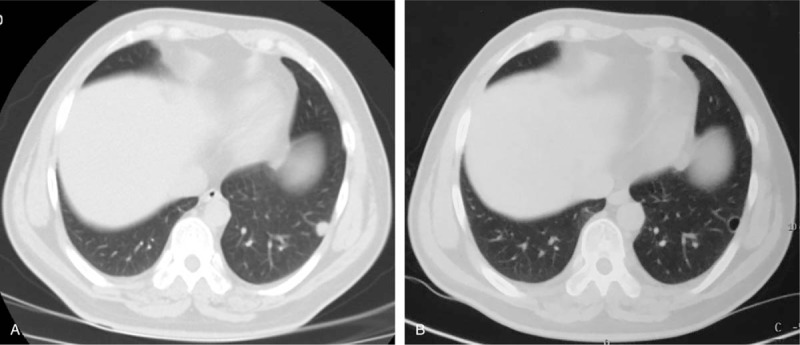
The chest CT shows the lesion replaced by a vacuole after apatinib treatment for 2 months.
